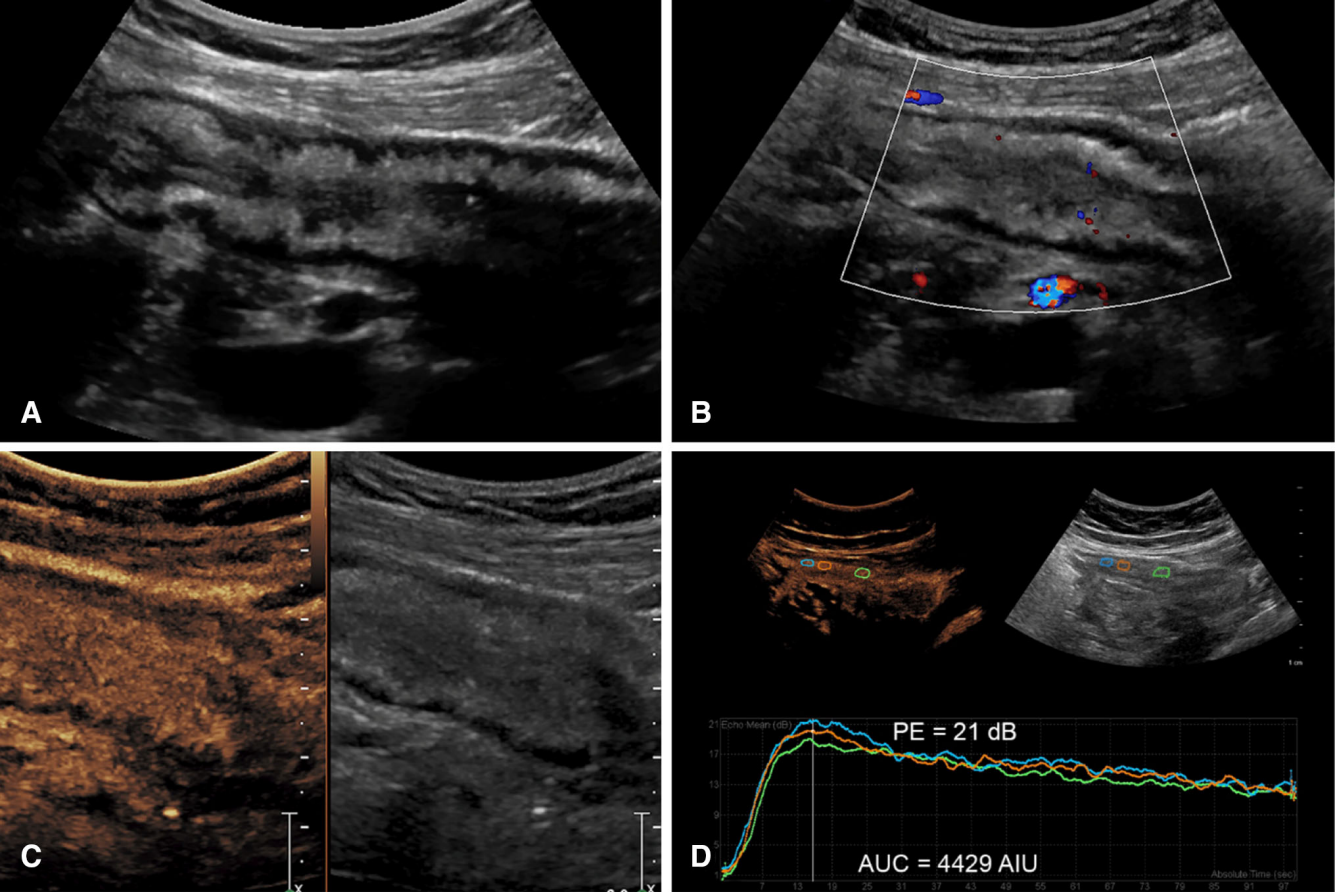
Indeterminate activity assessment resolved by CEUS in a 53-year-old female with Crohn’s. A Sagittal grayscale image shows moderate wall thickening of 7 mm. B CDI of the abnormal ileum does not show any color Doppler flow. Activity is therefore indeterminate. C CEUS shows transmural hyperenhancement. D CEUS and generated curve confirm moderate activity as suggested in image A and C. ROIs (color circles) are placed in the anterior bowel wall
